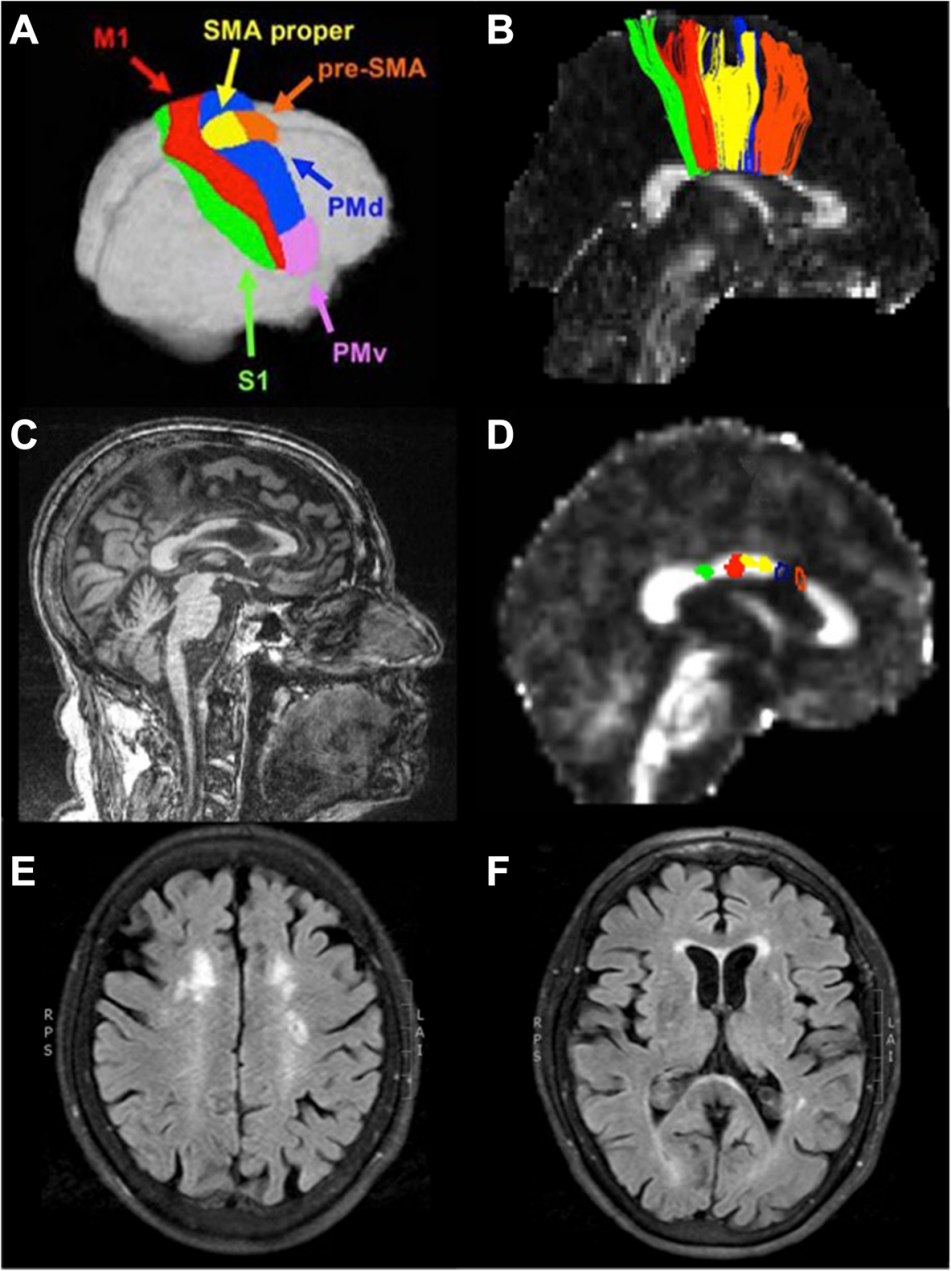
Diffusion tensor imaging: interhemispheric fiber tracts connecting principal, homologous sensorimotor cortical regions. Legend: a The Human Motor Area Template as defined by Mayka et al., 2006. b Interhemispheric fiber tracts from a healthy young adult, color-coded to match the cortical regions shown in panel A, taken from Fling et al., 2013. c Patient’s T1-weighted structural MRI showing an infarct within the anterior body of the corpus callosum. d Patient’s fractional anisotropy map, derived from diffusion weighted data. Fiber tracts were readily identified connecting the S1, M1, and SMA. No fiber tracts connecting either the PMd or the pre-SMA were identified; the blue and orange outlines demonstrate the location in the callosum where these fiber tracts would be expected to cross. e & f White matter hyperintensities within bilateral anterior periventricular regions. S1 = primary somatosensory cortex; M1 = primary motor cortex; SMA = supplementary motor area; PMd = dorsal premotor cortex; PMv = ventral premotor cortex
